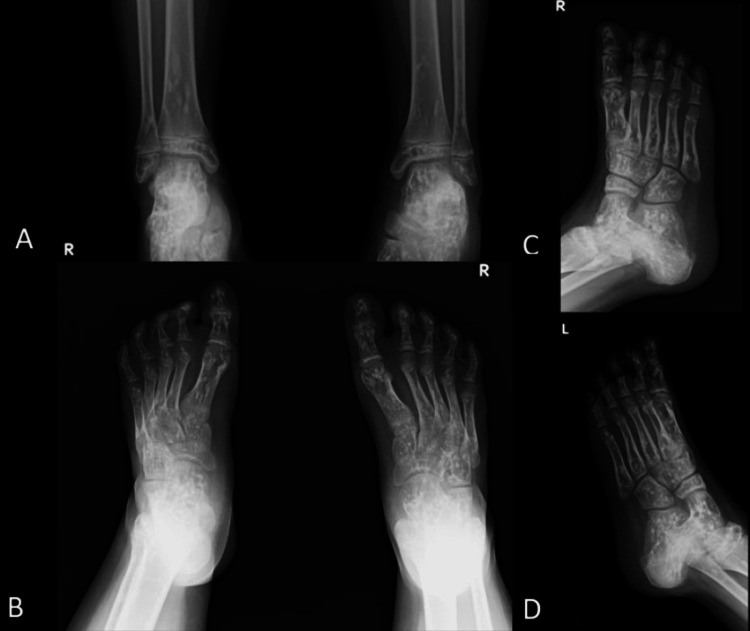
Plain radiographs of the son, a 14-year-old boy, show multiple homogeneous sclerotic lesions on the ankles and feet. (A) Anteroposterior view of ankles; (B) Anteroposterior view of feet; (C) Oblique view of right foot; and (D) Oblique view of left foot.
